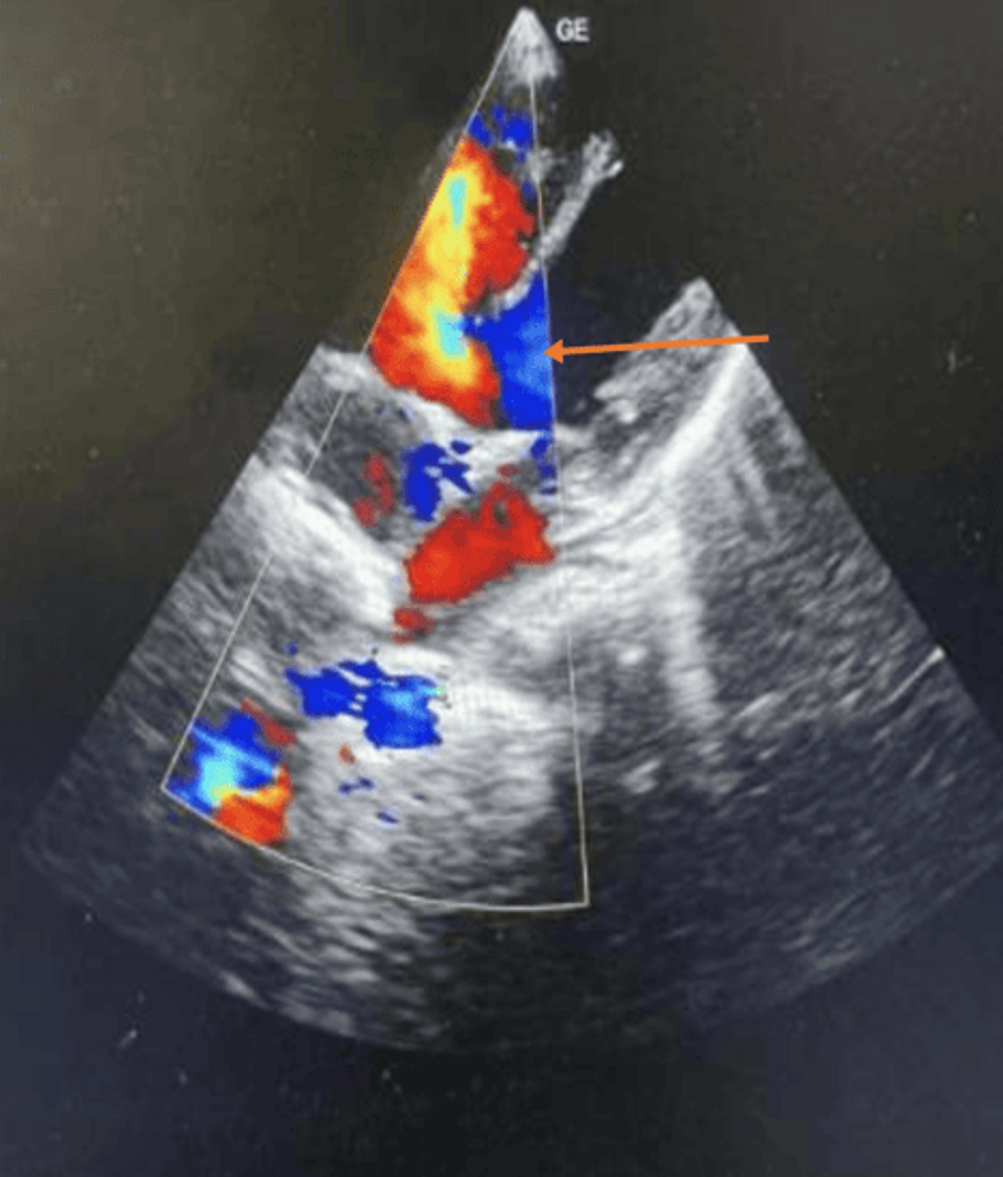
Echocardiography shows a large sub-aortic ventricular septal defect (VSD) in four chamber view.
